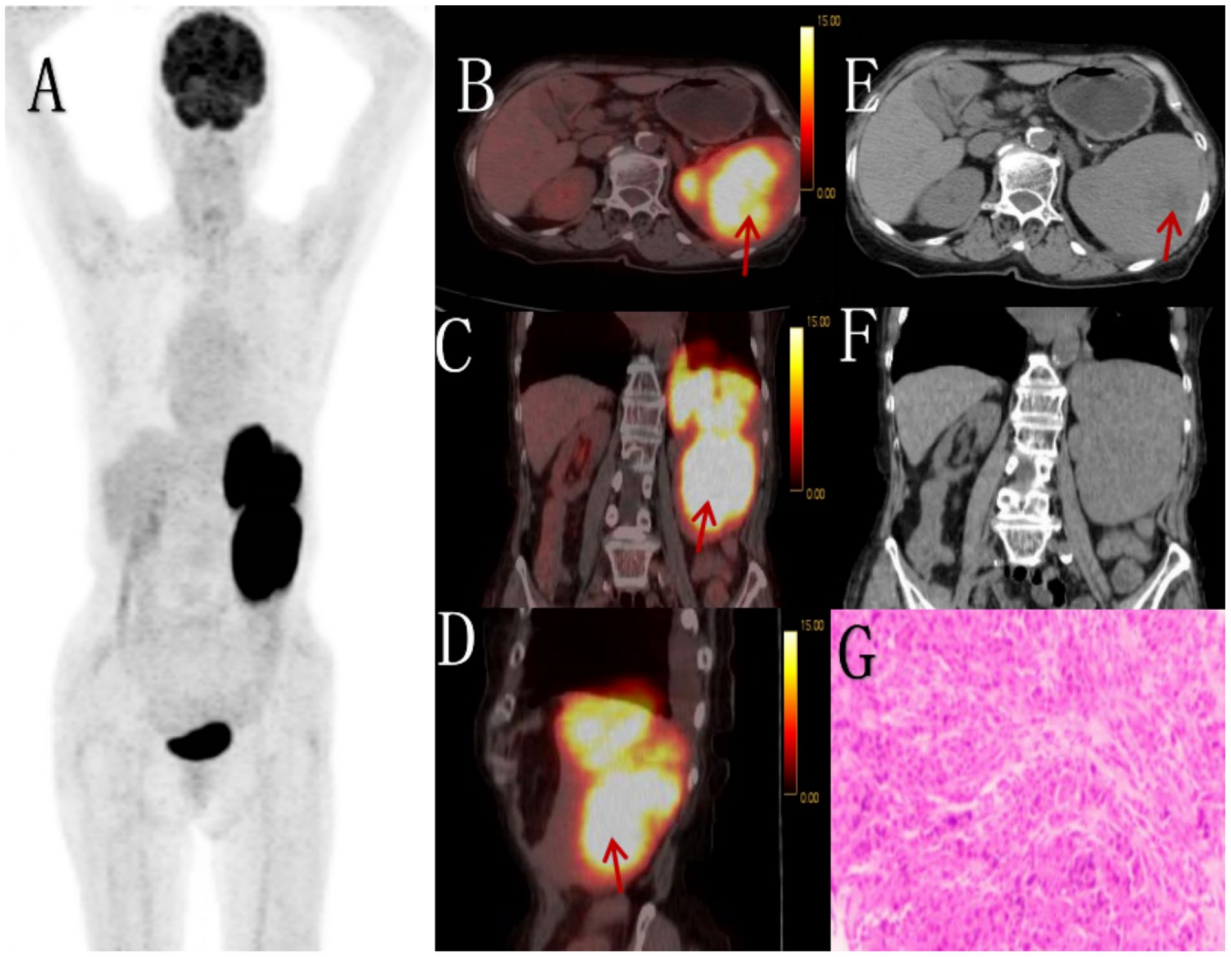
Female, 85 years old, diffuse large B-cell lymphoma. (A) whole body MIP, (B) axial fusion, (C) coronal fusion, (D) sagittal fusion, (E) axial CT, (F) coronal CT, (G) H-E × 200. 18F-FDG PET/CT demonstrated splenomegaly with an irregular soft tissue density mass (maximum cross-sectional dimension 17.7 × 8.5 cm) showing heterogeneous density and small hypodense necrotic areas. The lesion exhibited uniformly increased radiotracer uptake with a maximum standardized uptake value (SUVmax) of 14.4 (arrow, B–D). CT demonstrates an enlarged spleen with heterogeneous attenuation, showing multiple nodular and small patchy hypodense lesions. On contrast-enhanced scans, the spleen exhibits mild enhancement, while the necrotic areas show no significant enhancement (E). PET/CT-guided splenic biopsy confirmed the diagnosis of diffuse large B-cell lymphoma, non-germinal center subtype (G).
